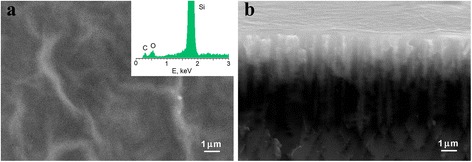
SEM images of the surface (a) and cross sections (b) of the PS–GO structures. *Inset*: X-ray surface microanalysis of the PS–GO structures
